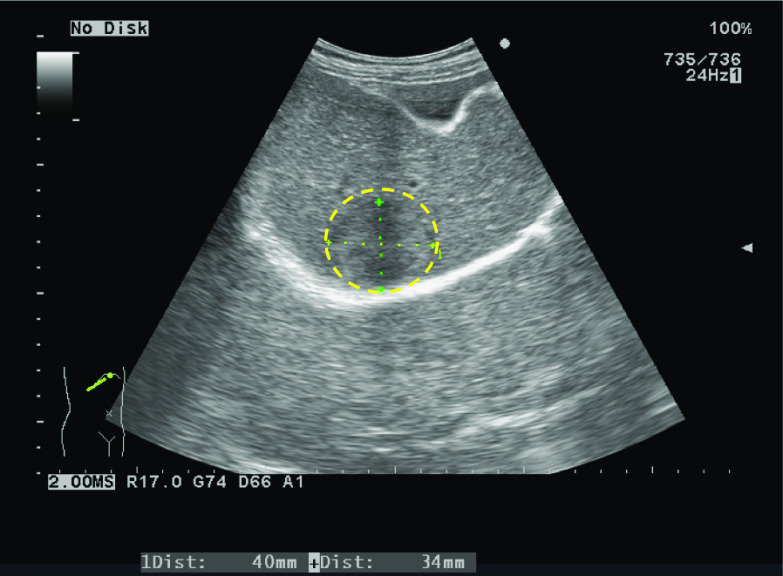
Abdominal ultrasonography (US). Abdominal US showed a 3-cm solid tumor in liver segment 8 with a heterogeneous irregular center and no fibrous capsule (dotted circle)
